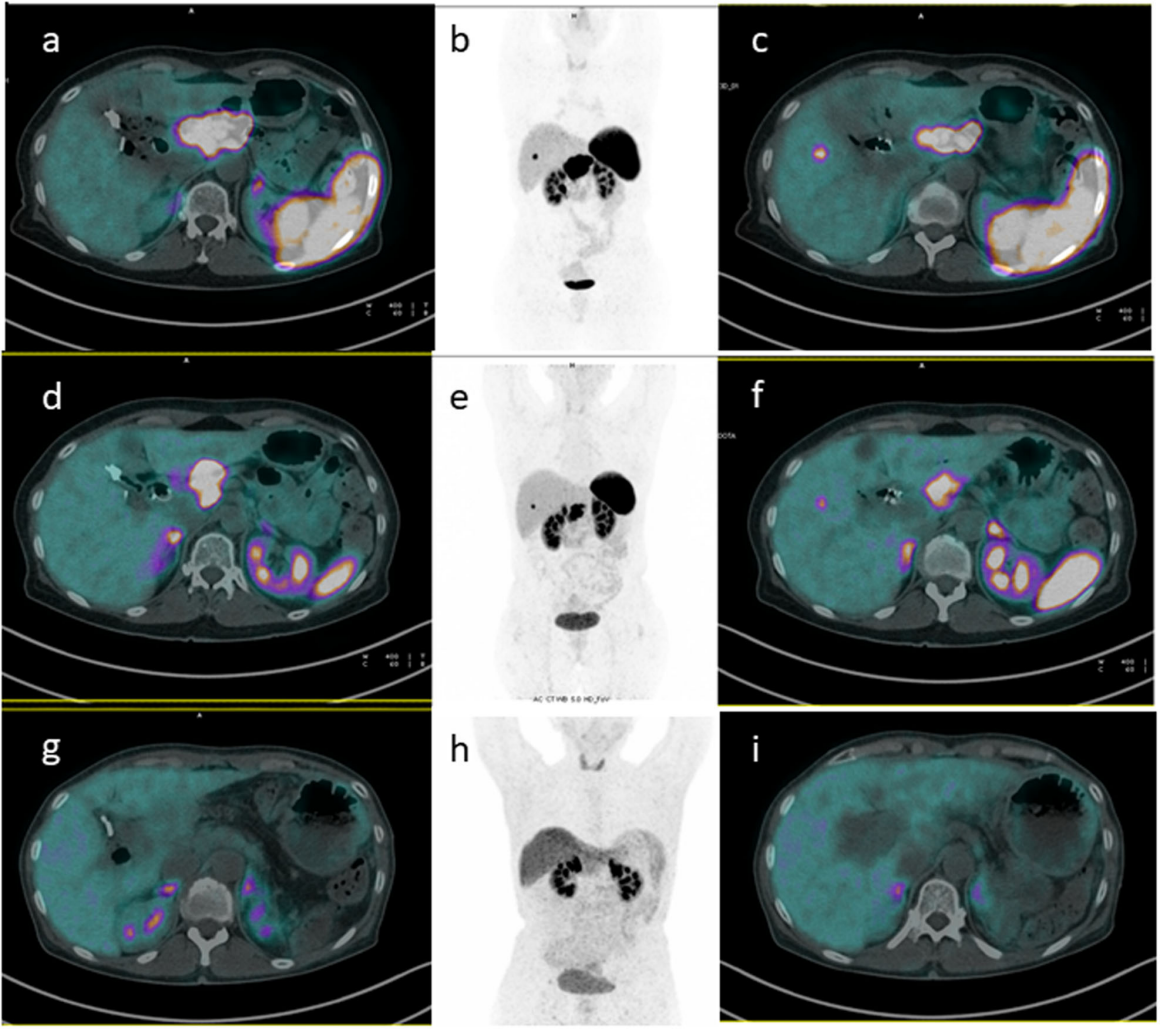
A successful case of neoadjuvant RLT. a–c Baseline [^68^Ga]-DOTATOC PET/CT of a patient with a primary NET mass of the pancreatic head (SUVmax 72) and a liver metastasis (SUVmax 24). d–f [^68^Ga]-DOTATOC PET/CT examination after V cycles of ^90^Y- and ^177^Lu RLT, showed a receptor density and dimensional reduction in pancreatic mass (SUVmax 56) and hepatic lesion (SUVmax 17). The patient then underwent second-line surgery. g–i [^68^Ga]-DOTATOC PET/CT scan one year after surgery well demonstrated a complete macroscopic response, with no pathological uptake
